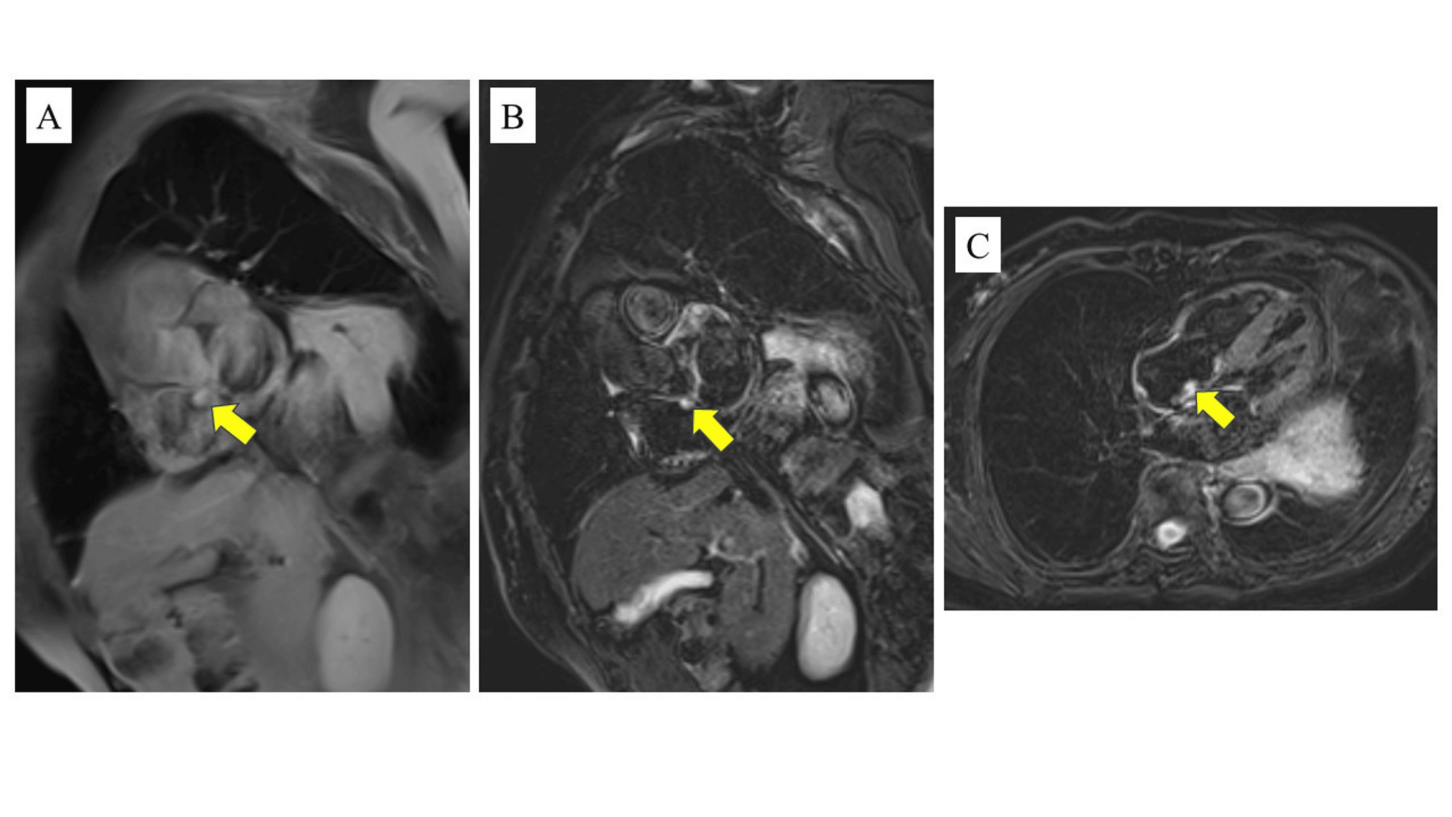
Preoperative CMR imaging CMR imaging revealed isointensity (yellow arrow) upon T1-weighted imaging (A), high signal intensity (yellow arrow) upon T2-STIR (B), and distinct enhancement (yellow arrow) after contrast administration (C). CMR: cardiac magnetic resonance, STIR: short tau inversion recovery.
